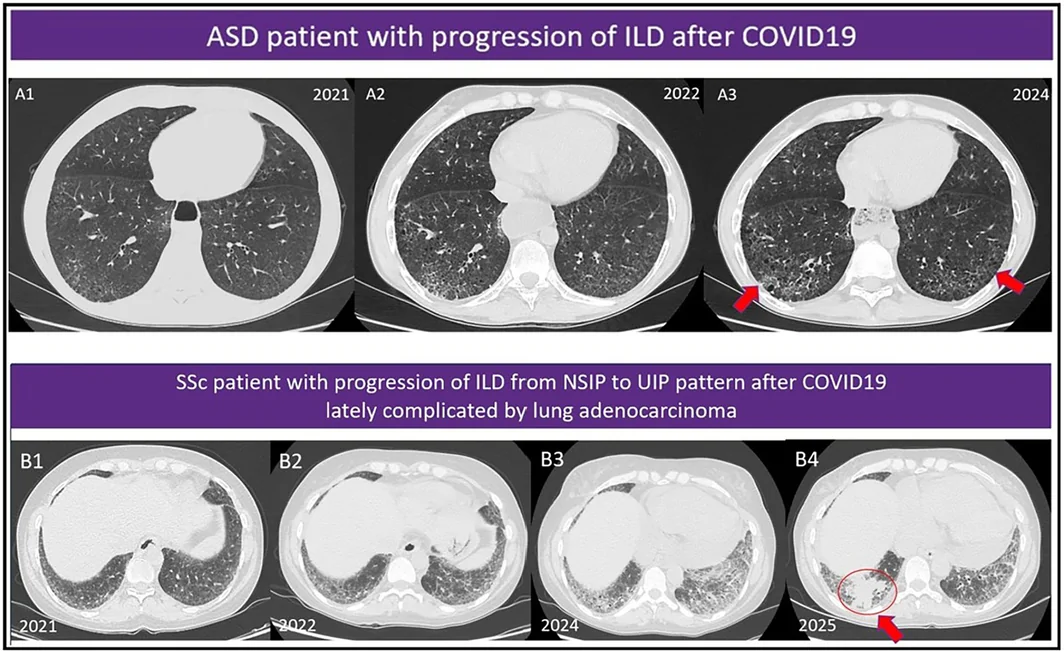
(A) SSc patient with progression of ILD after COVID‐19 (patient no. 20, Table 1). Before COVID‐19 (A1) mild dyspnea at exertion: at HRCT subtle GGO and fine reticular pattern in subpleural areas of the inferior lobes that involve < 20% of the lungs (fibrotic NSIP). Initial treatment with mycophenolate mofetil. The clinical, functional and radiological condition remained stable until 2021. After COVID‐19 (A2) worsening of cutaneous, vascular and pulmonary status, with increase, at HRCT, of interstitial thickening and more evident reticular opacities that involve > 20% of the lungs, in addition to appearance of traction bronchiolectasis. In May 2022 treatment with nintedanib was started. At the end of the follow‐up (2024, A3) dyspnea at rest with onset of cardiac involvement, tocilizumab was added to the regimen in February 2024. At HRCT, widespread areas of reticular thickening of the interstitium on a fibrotic basis, traction bronchiolectasis and distortion of the interstitial web with cystic changes with initial honeycombing pattern (red arrows). These findings, dominant in the subpleural and basal regions, are compatible with UIP pattern. (B) SSc patient with progression of ILD from NSIP to UIP pattern after COVID‐19 lately complicated by lung adenocarcinoma (patient no. 6, Table 1). Before COVID‐19 (B1), patient clinically asymptomatic with stable HRCT findings since 2013, namely subtle ground‐glass opacities (GGO) and fine reticular opacities in subpleural areas with basal predominance (mild ILD—NSIP pattern). After the first episode of COVID‐19 (B2), dyspnea at exertion, cough and HRCT scan showing an increase of GGO and interstitial reticular thickening in addition to development of subtle traction bronchiolectasis. After the second episode of COVID‐19 (B3), dyspnea at rest, cough and recurrent fever with further increase of interstitial thickening and extension of GGO at HRCT (prevalence of basal subpleural region, suggestive of UIP pattern): the clinical picture stabilises after a 6‐month therapy with nintedanib. In January 2025 (B4), worsening of clinical conditions and appearance of a nodular consolidation in the right lower lobe, which was found to be lung adenocarcinoma on transthoracic biopsy (red arrow).
